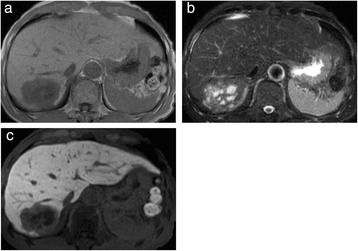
Magnetic resonance imaging (MRI). (a) T1-weighted image, (b) T2-weighted image, and (c) ethoxybenzyl (EOB)-MRI on the hepatobiliary phase. The tumor had low-signal intensity on T1-weighted and some high-signal intensity nodules in the tumor on T2-weighted images. EOB-MRI showed no uptake in the corresponding area on the hepatobiliary phase.
